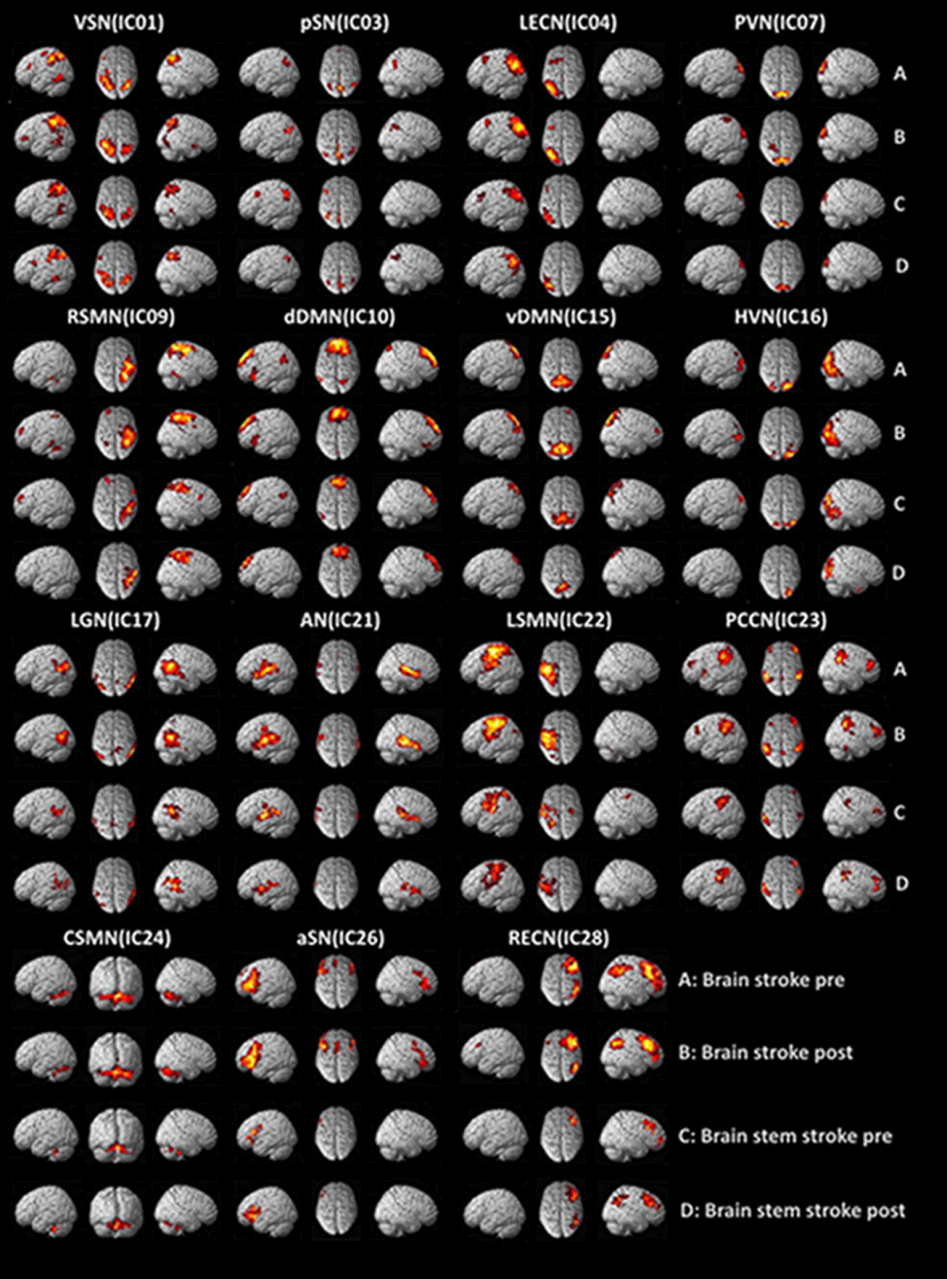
Functional MRI findings in stroke patients with dysphagia before and after swallowing training. VSN, visuospatial network; pSN, posterior salience network; LECN, left executive control network; PVN, primary visual network; RSMN, right sensorimotor network; dDMN, dorsal default mode network; vDMN, ventral default mode network; HVN, high visual network; LGN, language network; AN, auditory network; LSMN, left sensorimotor network; PCCN, precuneus network; CSMN, cerebellar sensorimotor network; aSN, anterior salience network; RECN, right executive control network.
